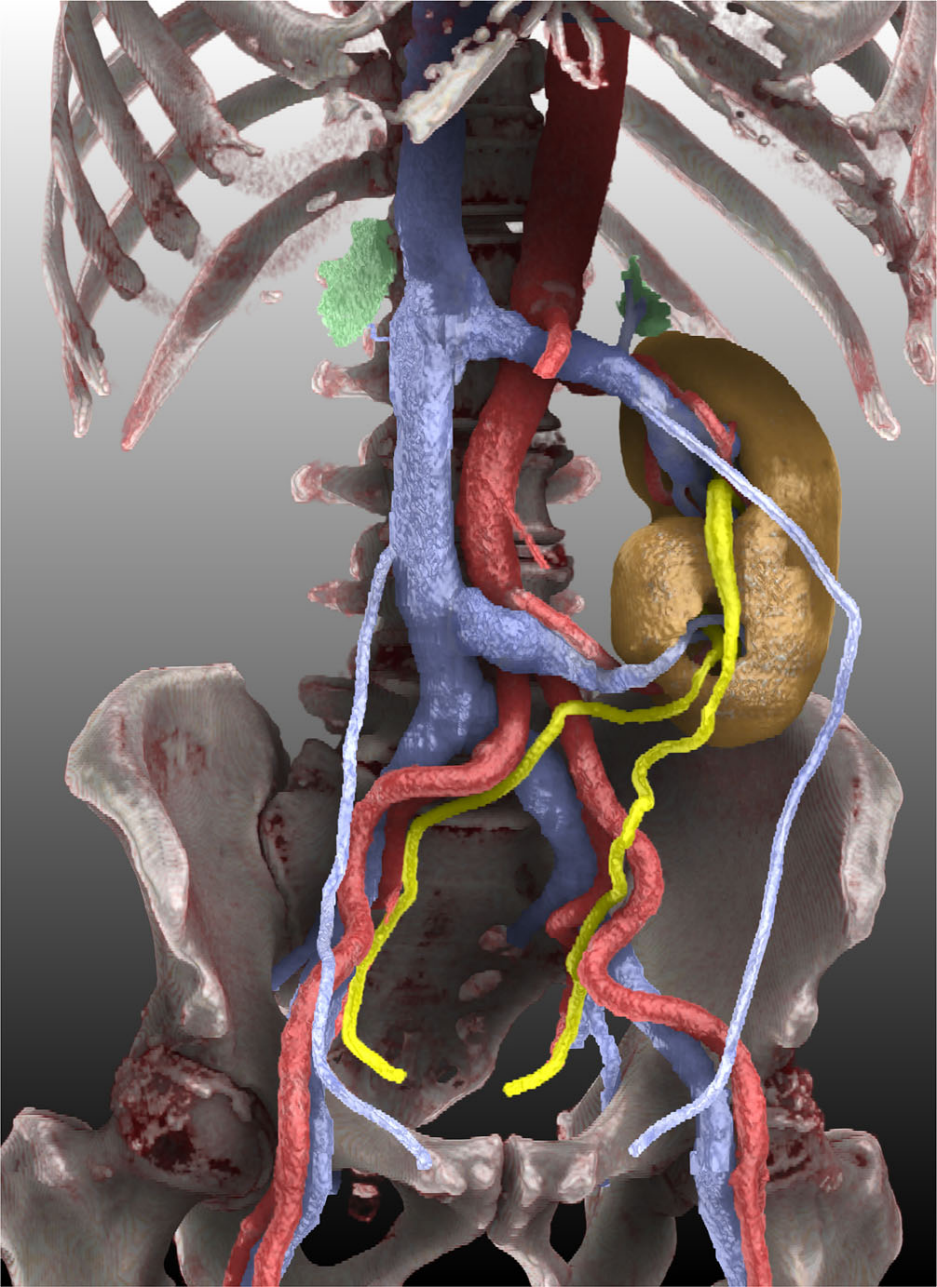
Cinematic rendering of a crossed fused renal ectopia and surrounding organs, incidentally discovered on a CT scan performed for metastatic screening in a 72‐year‐old man with no significant past medical history and newly diagnosed prostate cancer. The fused renal parenchyma is depicted in ocher, arteries in red, veins (including bilateral gonadal veins) in blue, both ureters in lemon yellow, bilateral adrenal glands in light green and bones in white and reddish brown, respectively.
